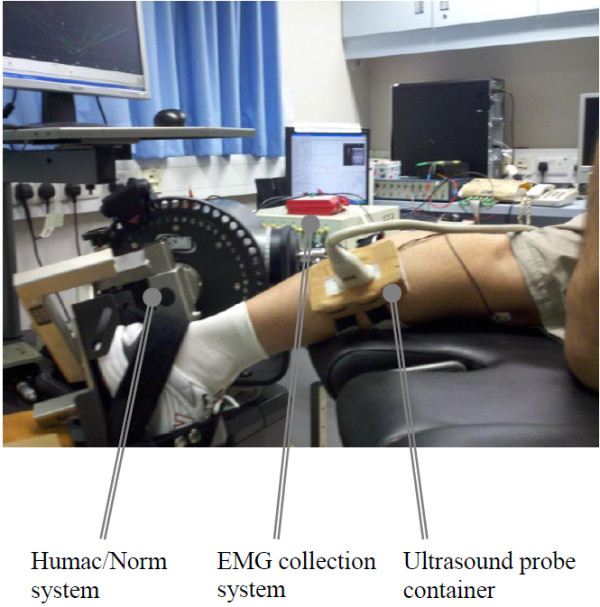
The experimental setup. The experimental setup including the torque, EMG and ultrasound image data collection modules.
